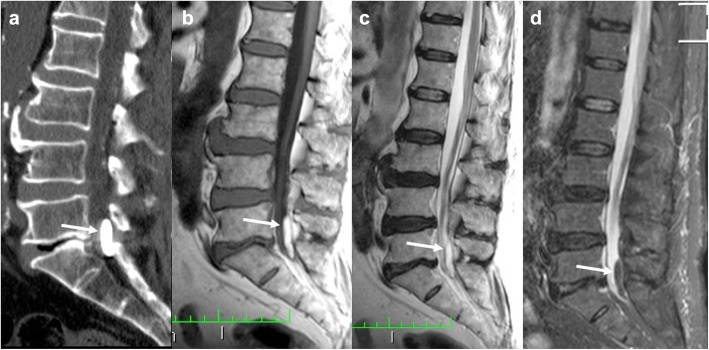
Lipiodol-induced adhesive arachnoiditis. Sagittal unenhanced CT (a), T1-weighted (b), T2-weighted (c), and STIR-weighted (d) images showing a mass in the spinal canal at the level of L5-S1 encasing the corresponding nerve roots. It appears hyperdense on CT and hyperintense on both T1- and T2-weighted sequences with signal suppression on STIR (arrows). The cauda nerve roots appear adherent to each other, consistent with the diagnosis of arachnoiditis
